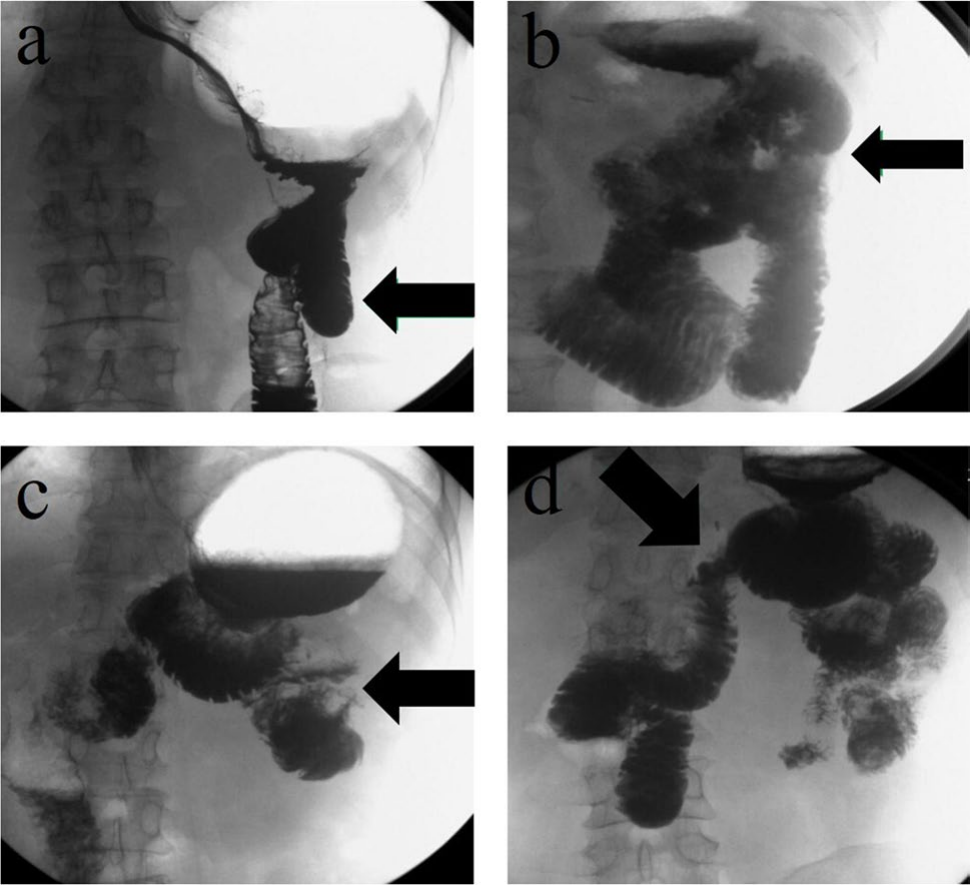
Upper gastrointestinal radiography after Uncut Roux-en-Y anastomosis. Upper gastrointestinal radiography showing the closure of the afferent limb (black arrows) 6 months after the operation (a) and recanalization (black arrow) 9 months after the operation (b). In another case, upper gastrointestinal radiography showing the closure of the afferent limb (black arrow) 9 months after the operation (c) and recanalization (black arrow) 12 months after the operation (d)
